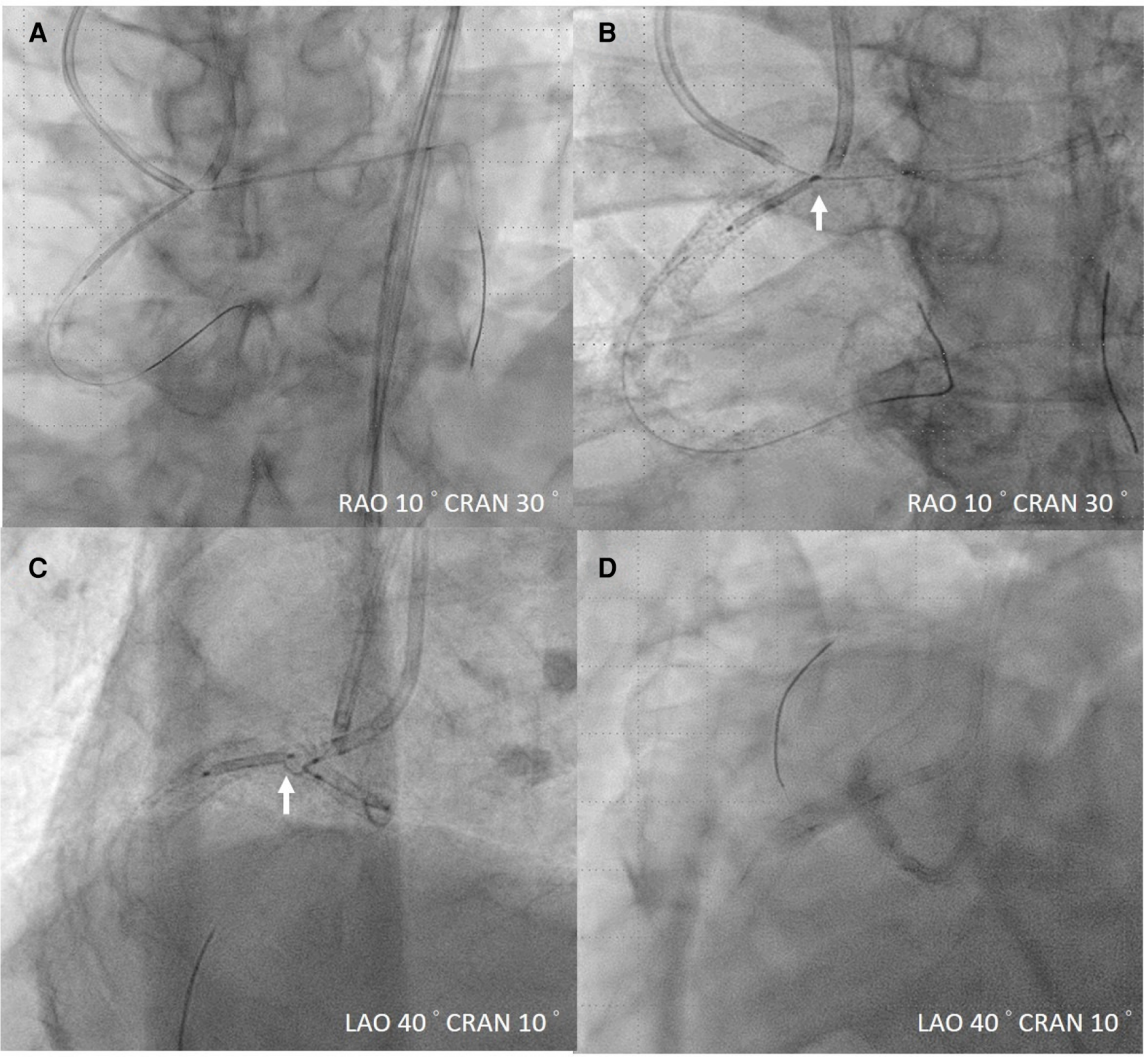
Management of AAOCA stenosis. (A) Intravascular ultrasound (IVUS)-guided stent deployment. (B,C) White arrow indicates the proximal mark of the right coronary artery stent, positioned close to the center of the proximal left main coronary artery stent, in accordance with IVUS guidance. (D) Kissing balloon technique for ostium bifurcation. AAPCA, anomalous aortic origin of a coronary artery.
